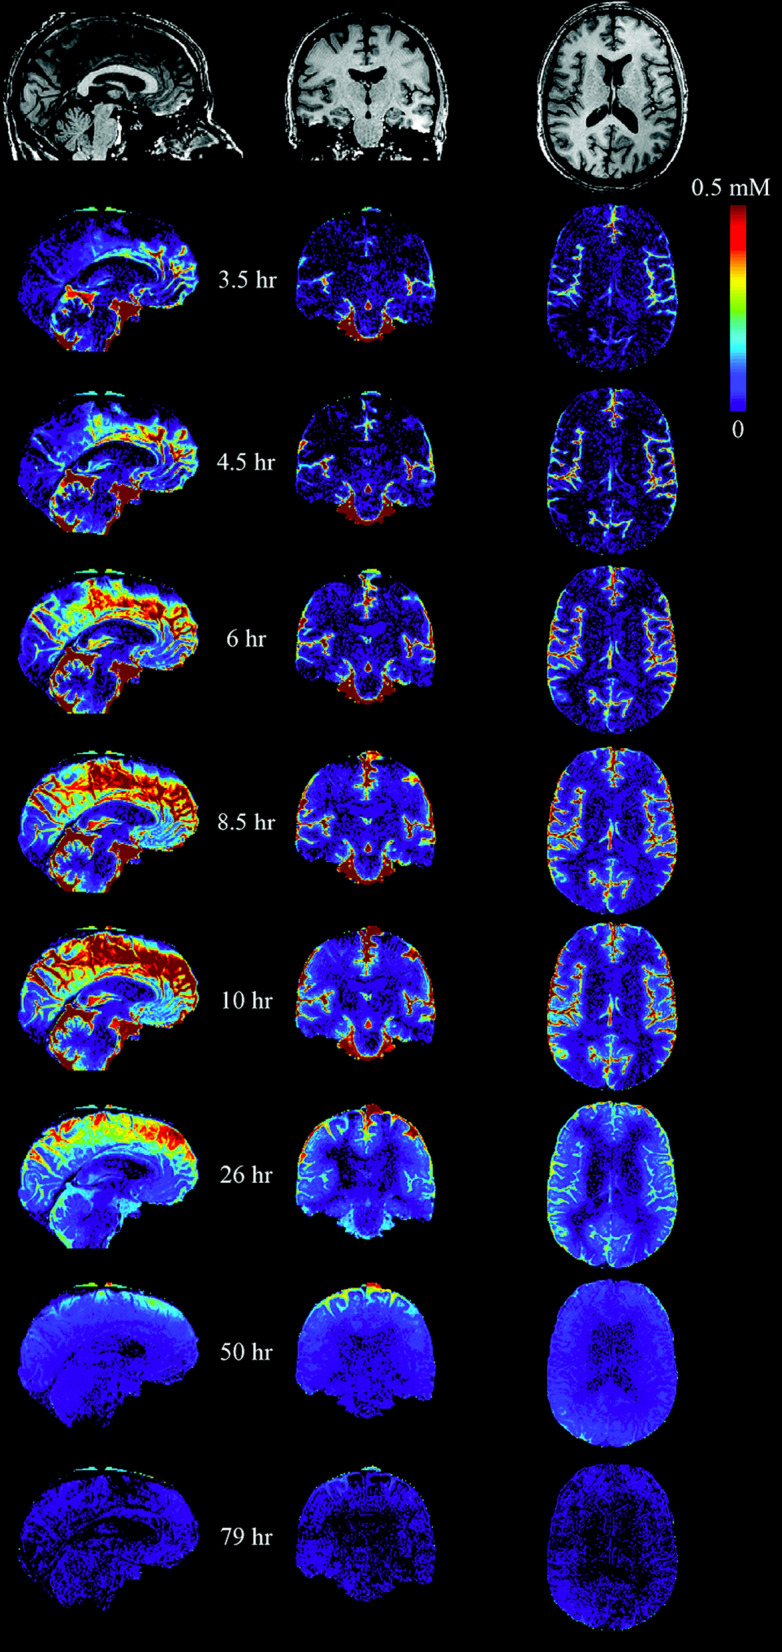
Human MRI images showing changes in gadobutrol concentration following an intrathecal injection. At *t *= 0, 1 µmol of gadobutrol was injected into the subarachnoid space of the spinal cord. It reached the cisterna magna in about 3 h and spread over the surface of the brain in the next 7-8 h. Note that the gadobutrol concentrations on the dorsal surface of the brain persist for longer than in the basal cisterns as expected if elimination of gadobutrol occurs primarily from the cisterns, e.g. across the cribriform plate, but not from the dorsal subarachnoid spaces. The partial analysis of these data in [117] shows that in various regions of the brain the concentrations in grey matter are still increasing up to 12 h and subsequently decrease over days in parallel with that in CSF adjacent to the region. However, even at their maximum they are less (2 to 5 fold depending on region) than the concentrations that were achieved in the adjacent subarachnoid spaces. At the very latest times, the concentrations in parenchyma appear to exceed the then current concentrations in adjacent subarachnoid spaces. The subject was awake from the time of administration until after the scan at 10 h and had a normal nights sleep before each of the last three scans. Figure taken with permission from Watts et al., Am. J. Neuroradiol., 2019 [117]
